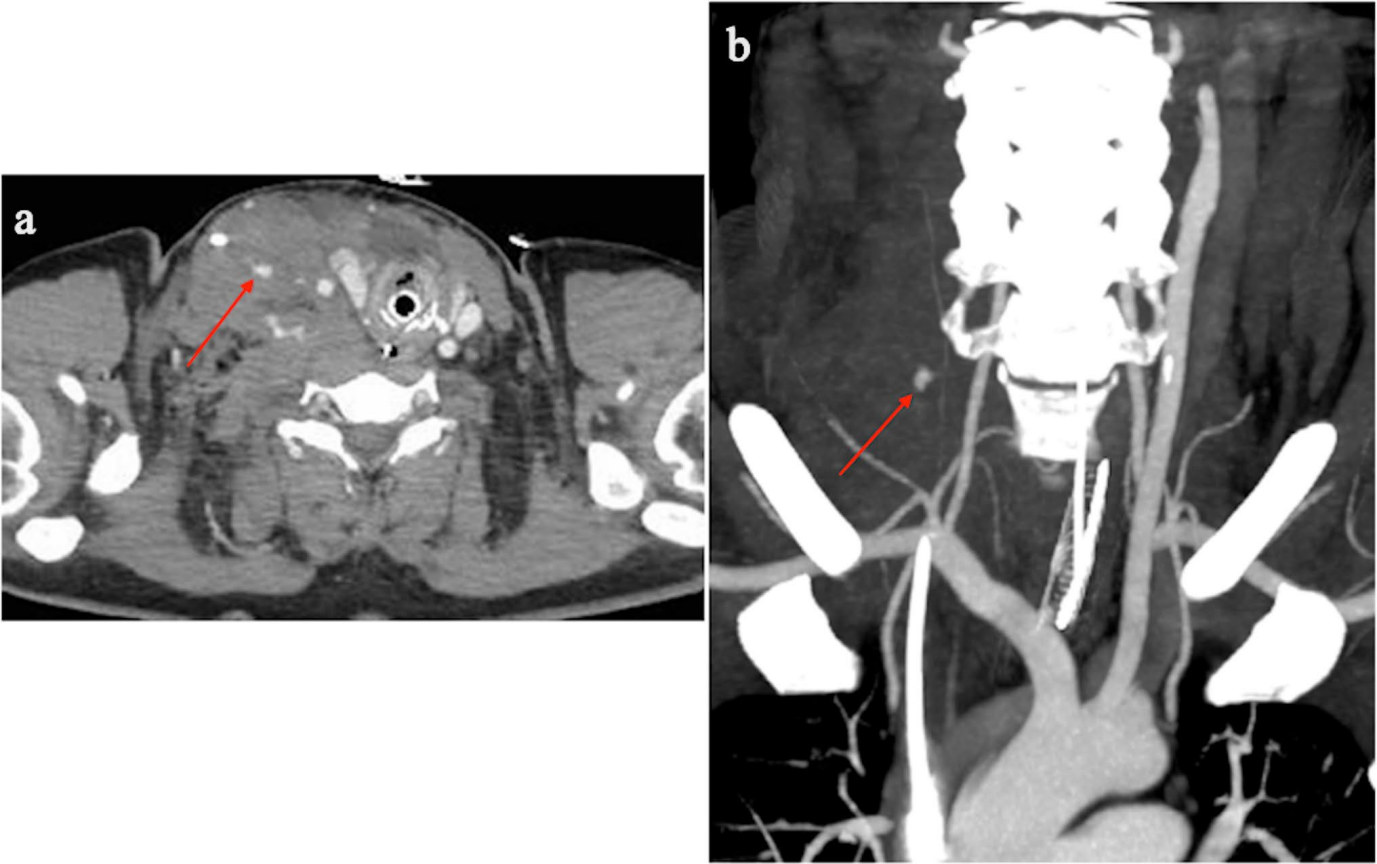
Contrast-enhanced CT showing active bleeding fed from branches of the right superior thyroid artery [a, axial image (arrow), b, maximum intensity projection (MIP) view (arrow)]
